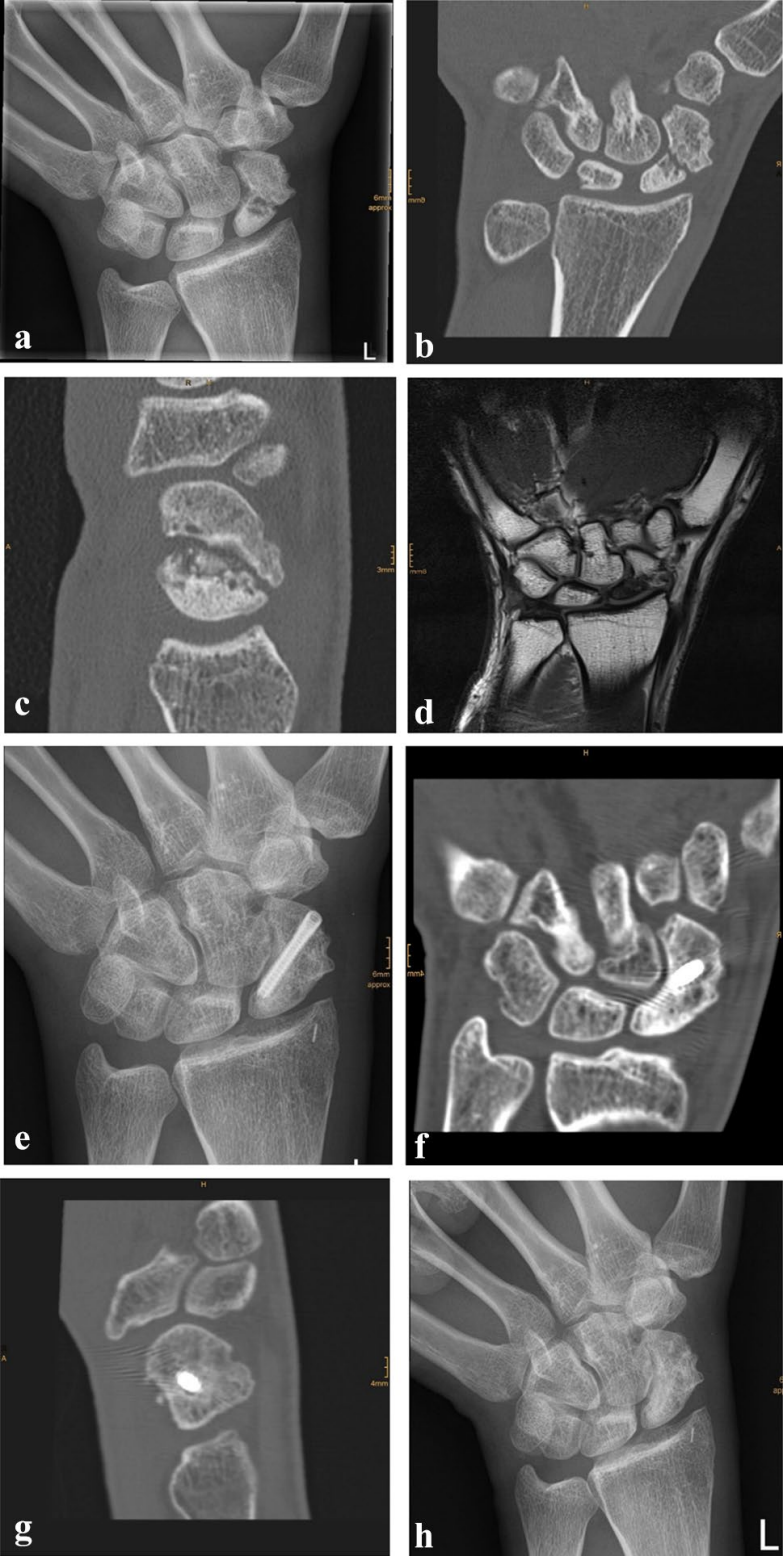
a Dorsopalmar radiograph of a 31-year-old man showing a non-union 3 years after sustaining a scaphoid fracture which initially remained undetected. b Coronal CT scan showing the scaphoid non-union in the mid-waist. c Sagittal CT scan. d Coronal gadolinium-enhanced MRI scan showing an avascular necrosis of the proximal pole. e Dorsopalmar radiograph 2 years after implantation and screw fixation of a vascularized bone graft from the medial femoral condyle showing complete healing with no signs of carpal malalignment. f Coronal CT scan showing complete healing. g Sagittal CT scan. h Dorsopalmar radiograph after removal of the screw (2.5 years after surgery) showing no signs of osteoarthritis or carpal collapse
